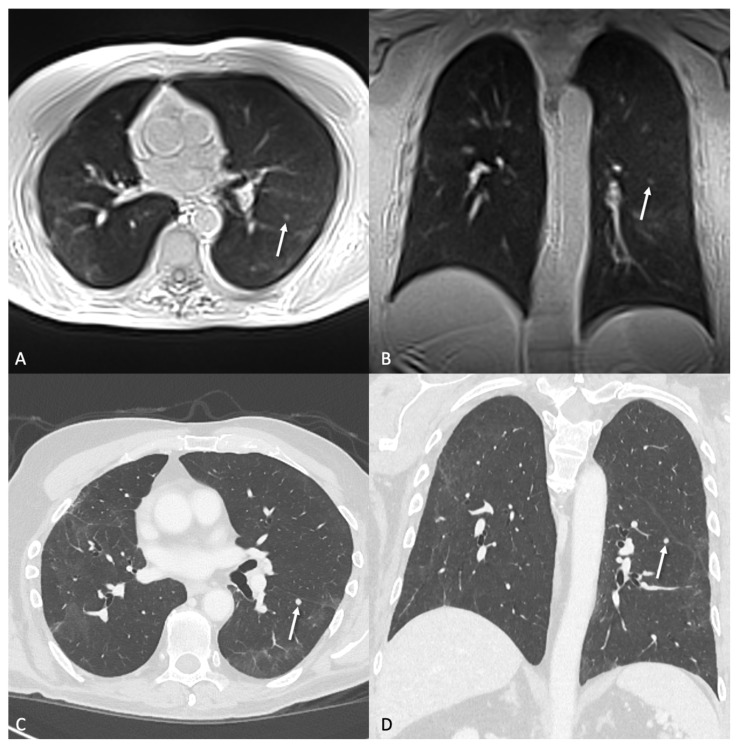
A 77-year-old patient with bipulmonary metastases of a malignant melanoma. The transversal (A) and coronal (B) ultra-short echo time (UTE) sequence clearly showed a 4.5 × 4.4 mm lung metastasis (arrow) in the left lower lobe. (C,D) depict the same nodule on CT with a 1 mm slice thickness, acquired on the same day.
